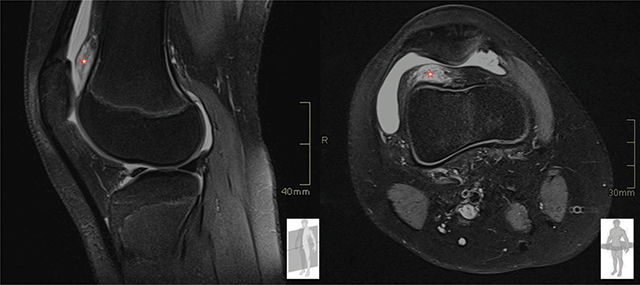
MRI, T2-weighter fat suppressed. PFP edema with mass-like protrusion into the suprapatellar pouch. Joint effusion.
